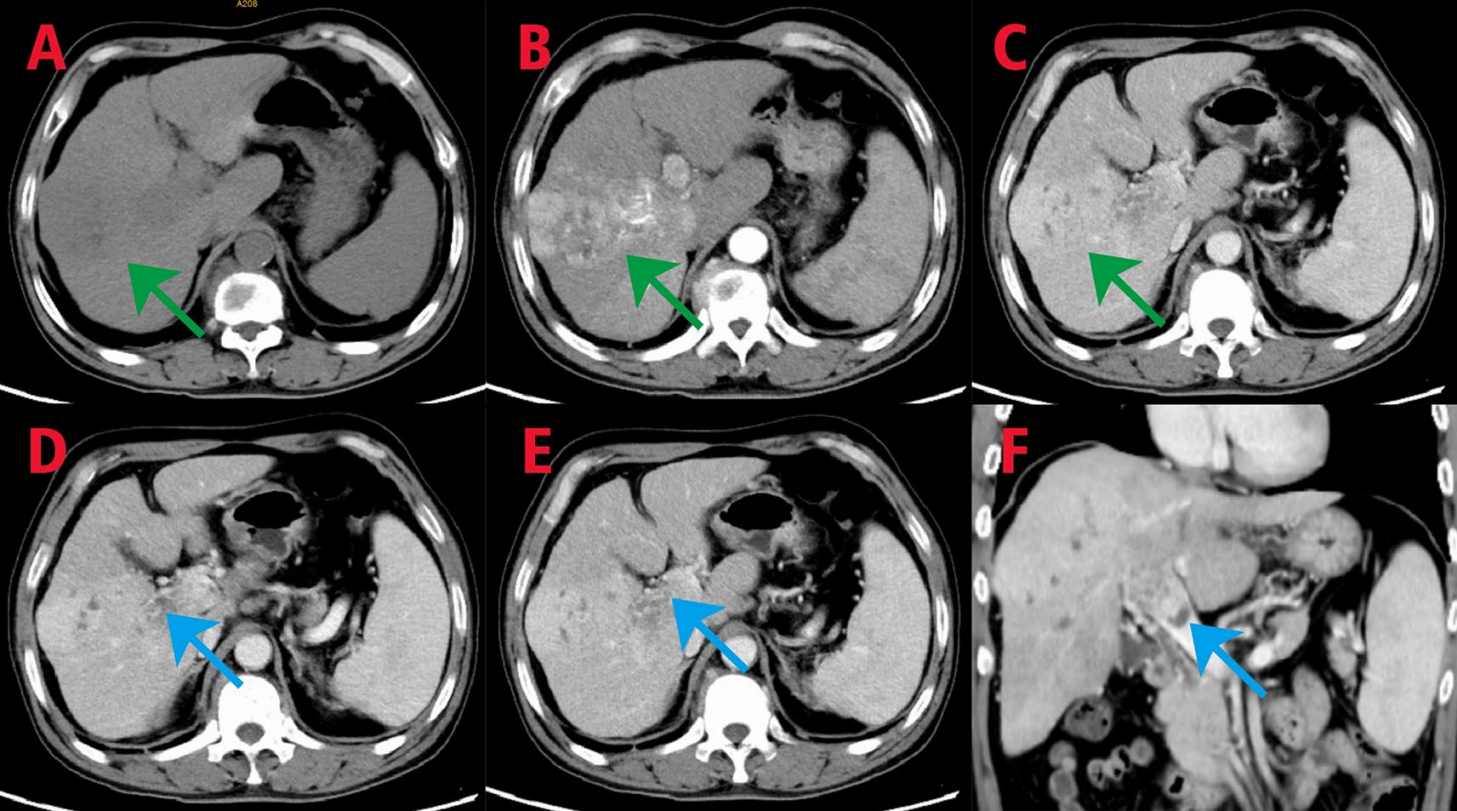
CT imaging findings of the patient upon admission. In the plain scan, a nodular low-density lesion with indistinct borders is observed in the right lobe of the liver A indicated by green arrows. The contrast-enhanced scan in the arterial phase shows patchy areas of obvious density and abnormal enhancement B indicated by green arrows, while the enhancement degree is reduced in the portal vein phase and delayed phase C indicated by green arrows. There is a patchy filling defect in the main trunk of the portal vein, which is enhanced on the contrast-enhanced scan D, E, F, indicated by blue arrows
